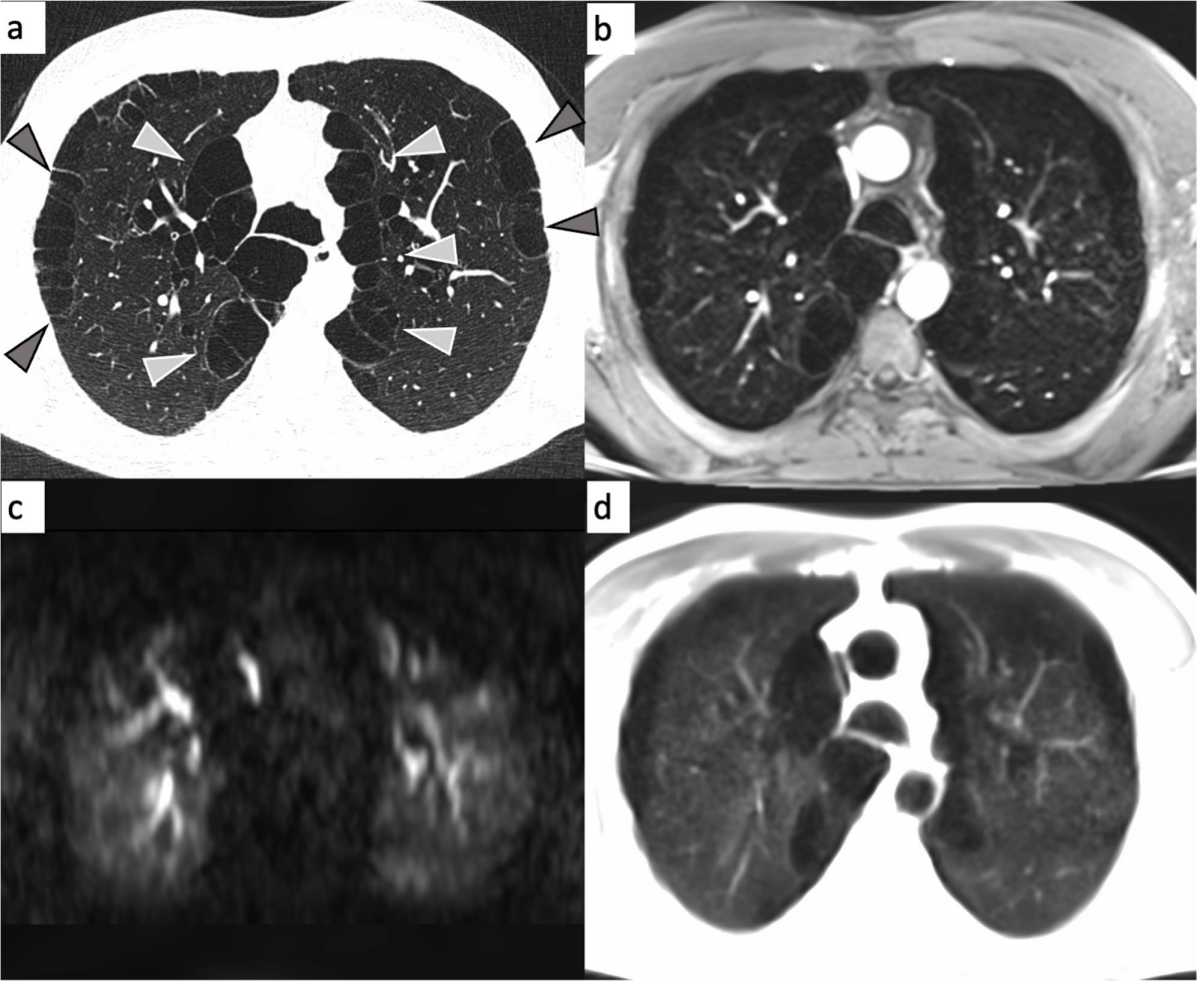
Paraseptal emphysema in a 51-year-old male participant (classified as airway predominant COPD phenotype). Arrowheads on the CT images (a) indicate areas of paraseptal emphysema with large bullae. On the corresponding MRI, the affected parts of the lung appear as lung parenchyma defects on the contrast-enhanced transverse 3D gradient echo image (b), as perfusion deficits in the DCE series (c) and as signal void in the transverse half Fourier fast spin echo images (d)
